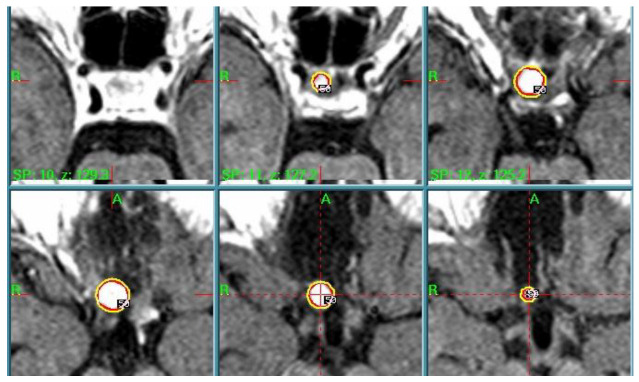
Case 5 treatment planning. The small lesion is identified in T1-weighted MRI (red line) and the 50% isodose line (yellow) is prescribed, showing the high conformation of the dose plan.
